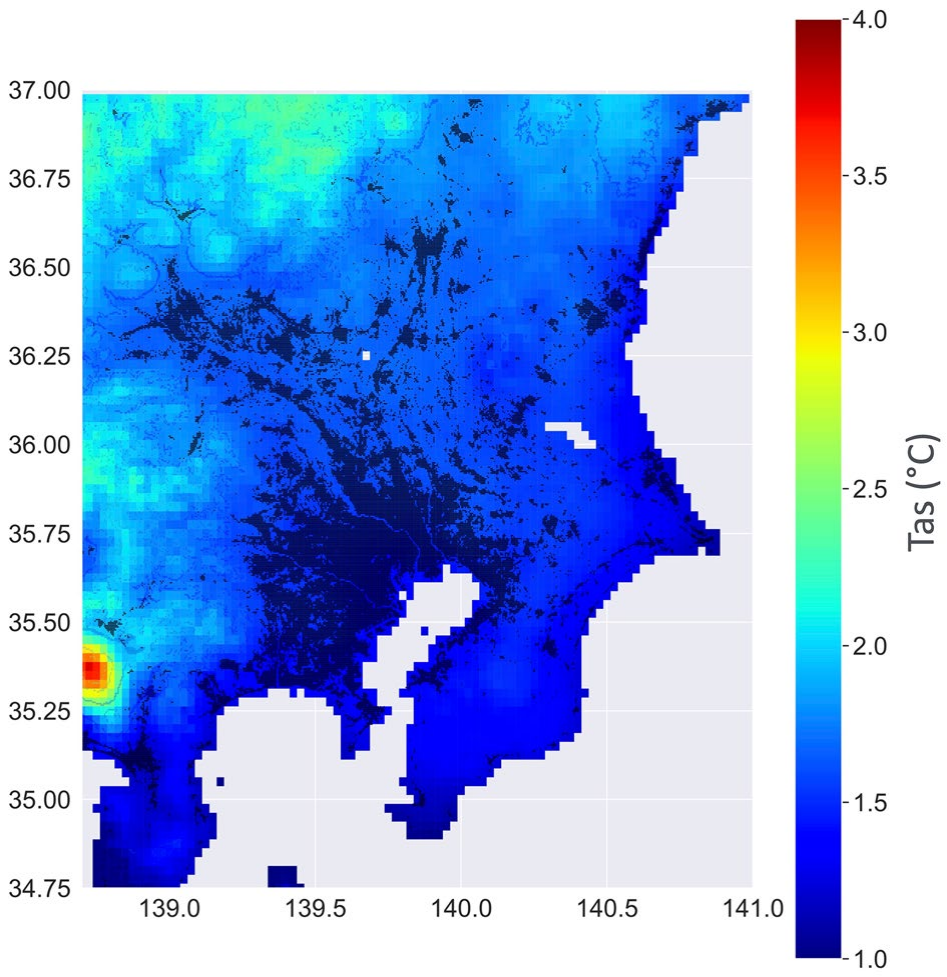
MRI time series differences for temperature (°C) as shown in Fig. 6a, except for winter (DJF) months. Mount Fuji’s location corresponds to the high patch in the southwest. Plots were generated using Python’s Matplotlib library, version 3.4.3, https://matplotlib.org/3.4.3/contents.html.
